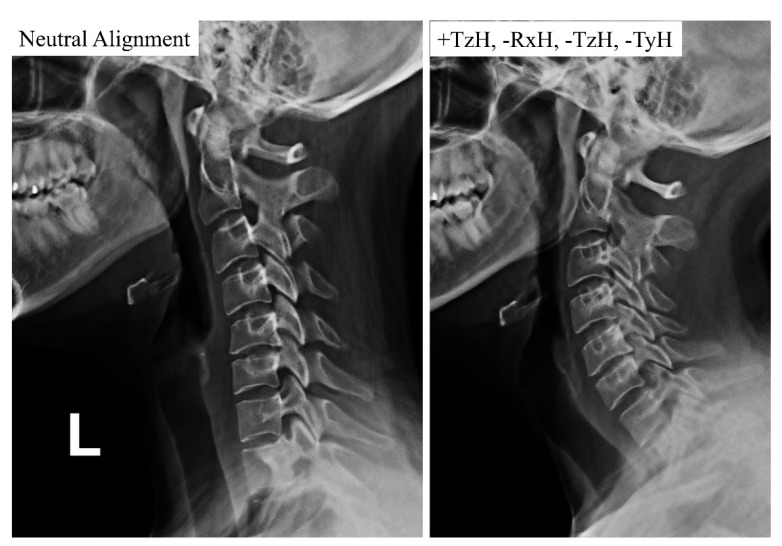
A patient’s lateral cervical x-rays are shown in neutral and after the mirror image exercise sequence (+TzH, −RxH, −TzH, −TyH) demonstrating the change in alignment from neutral with this sequence of movements: forward head posture (+TzH), upper neck/head extension (−RxH), followed by posterior head translation (−TzH) with an inferior compression component (−TyH). Images courtesy of Curtis Fedorchuk, reprinted with permission [28,29].
